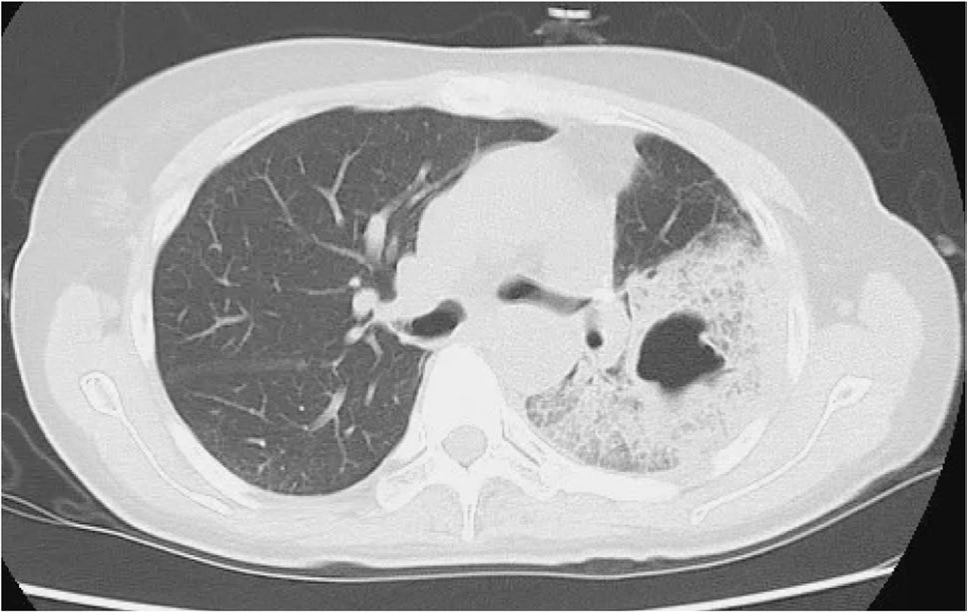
Chest computed tomography shows cavitation in the left lower lobe with surrounding infiltrates
